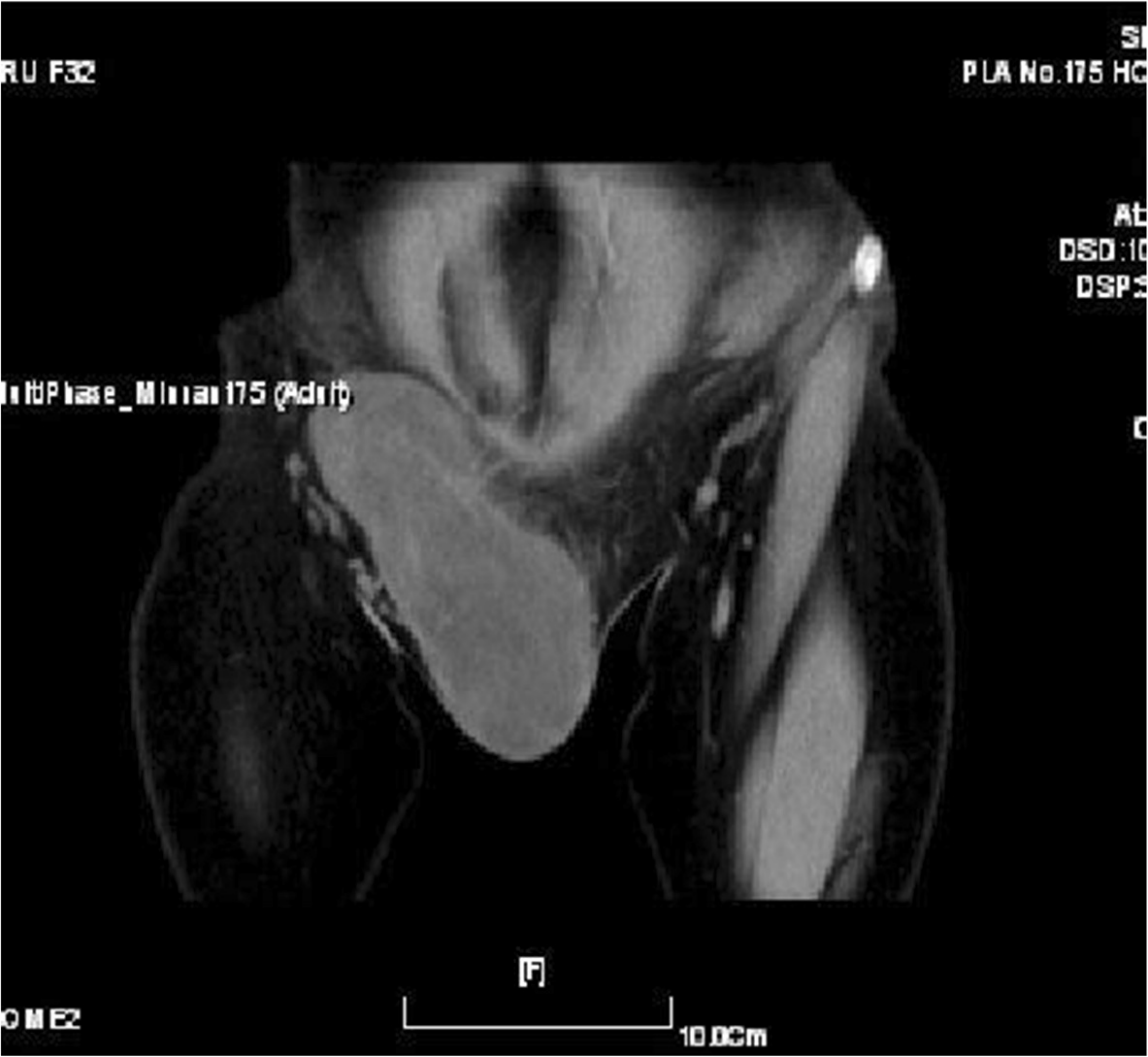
Computed tomography scan showed the tumor was located in the subcutis, well-demarcated, and isolated from the abdominal cavity.
